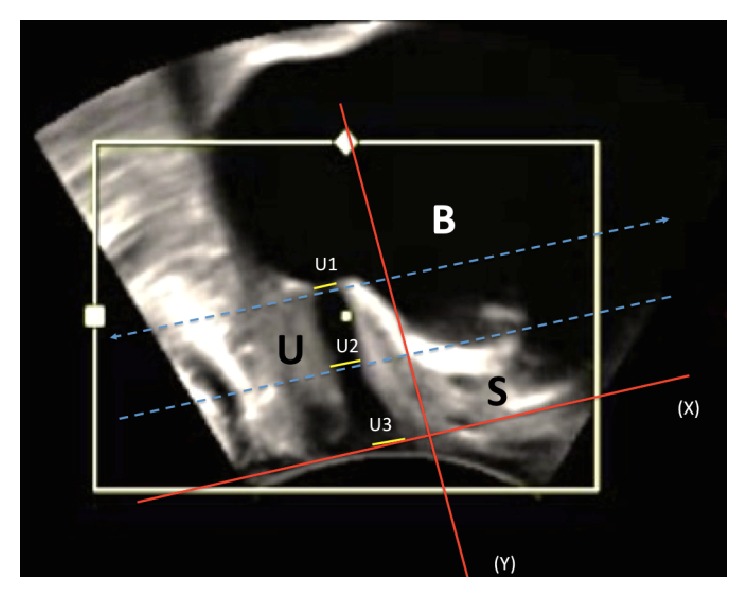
Perineal ultrasound image on midsagittal plane from a patient at rest. The positions of symphysis (S), urethra (U), bladder (B), reference line (X), the line orthogonal to reference line (Y), and urethral lumen (proximal (U1), medial (U2), and distal (U3)) are indicated.
